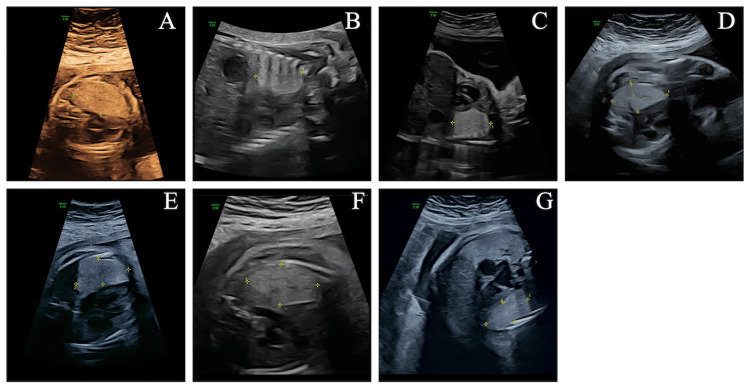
CPAM ultrasounds at various gestational ages A: Ultrasound demonstrating left-sided CPAM with CVR = 0.48 cm^2^ at 23 weeks of gestation. There is no evidence of fetal hydrops. Image obtained during Maternal Fetal Medicine evaluation at Florida Perinatal Associates, with patient consent. B: Ultrasound demonstrating left-sided CPAM with CVR = 0.51 cm^2^ at 24 weeks of gestation. There is no evidence of fetal hydrops. Image obtained during Maternal Fetal Medicine evaluation at Florida Perinatal Associates, with patient consent. C: Ultrasound demonstrating left-sided CPAM with CVR = 0.45 cm^2^ at 25 weeks of gestation. There is no evidence of fetal hydrops. Image obtained during routine Maternal Fetal Medicine evaluation at Florida Perinatal Associates, with patient consent. D: Ultrasound demonstrating left-sided CPAM with CVR = 0.63 cm^2^ at 26 weeks of gestation. There is no evidence of fetal hydrops. Image obtained during routine Maternal Fetal Medicine evaluation at Florida Perinatal Associates, with patient consent. E: Ultrasound demonstrating left-sided CPAM with CVR = 0.31 cm^2^ at 27 weeks of gestation. There is no evidence of fetal hydrops. Image obtained during routine Maternal Fetal Medicine evaluation at Florida Perinatal Associates, with patient consent. F: Ultrasound demonstrating left-sided CPAM with CVR = 0.33 cm^2^ at 28 weeks of gestation. There is no evidence of fetal hydrops. Image obtained during routine Maternal Fetal Medicine evaluation at Florida Perinatal Associates, with patient consent. G: Ultrasound demonstrating left-sided CPAM with CVR = 0.27 cm^2^ at 30 weeks of gestation. There is no evidence of fetal hydrops. Image obtained during routine Maternal Fetal Medicine evaluation at Florida Perinatal Associates, with patient consent.
